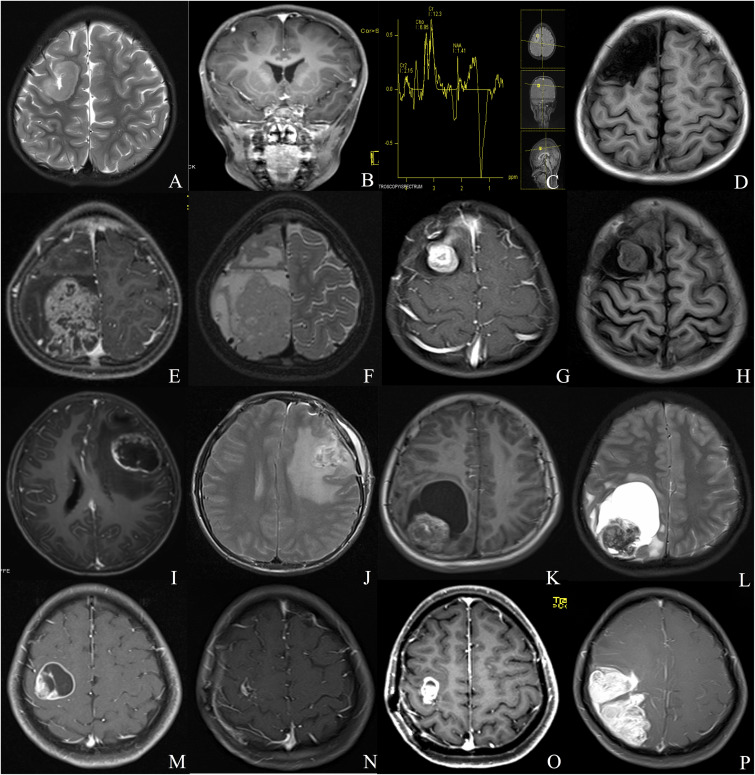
Different radiologic characteristics of CEs. Case 1 (A–D) The tumor was found in the right frontal cortex (A) and showed no obvious enhancement (B); MRS depicted increased choline and decreased *N*-acetyl-aspartate (C); postoperative MRI scan showed total resection of the tumor (D). Case 2 (E,F) A solid tumor with heterogeneous enhancement was depicted in the right parietal lobe. Case 3 (G,H) The tumor showed homogeneous enhanced solid appearance on MRI (G,H). Case 4 (I,J) The tumor depicted a rim-enhanced cystic lesion with obvious peritumoral edema. Case 5 (K,L) The tumor demonstrated a solid mural with peritumoral cyst. Case 6 (M–P) The lesion showed rim-enhanced cystic lesion, without peritumoral edema (M); subtotal resection was applied due to the central region location of the tumor (N); the tumor recurred 1 year after the surgery (O), and the lesion continued to grow during the follow-up (P).
